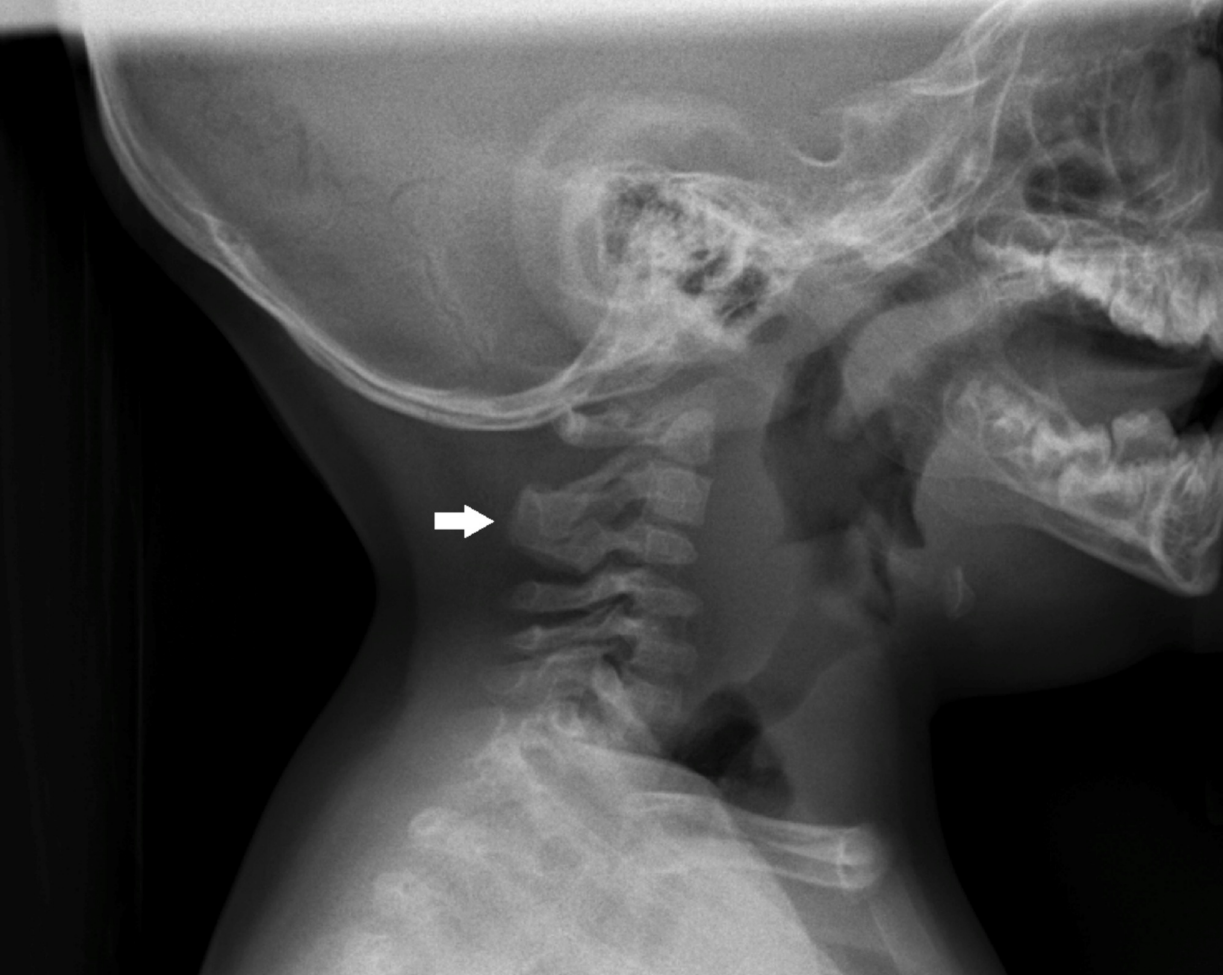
Klippel-Feil syndrome Lateral radiograph of the cervical region showing C2/C3 (white arrow) Klippel-Feil syndrome, a rare congenital disorder characterized by fusion of at least two cervical vertebrae resulting in restricted mobility of the upper neck. Of note, Klippel-Feil syndrome may have signs of basilar invagination [7].
